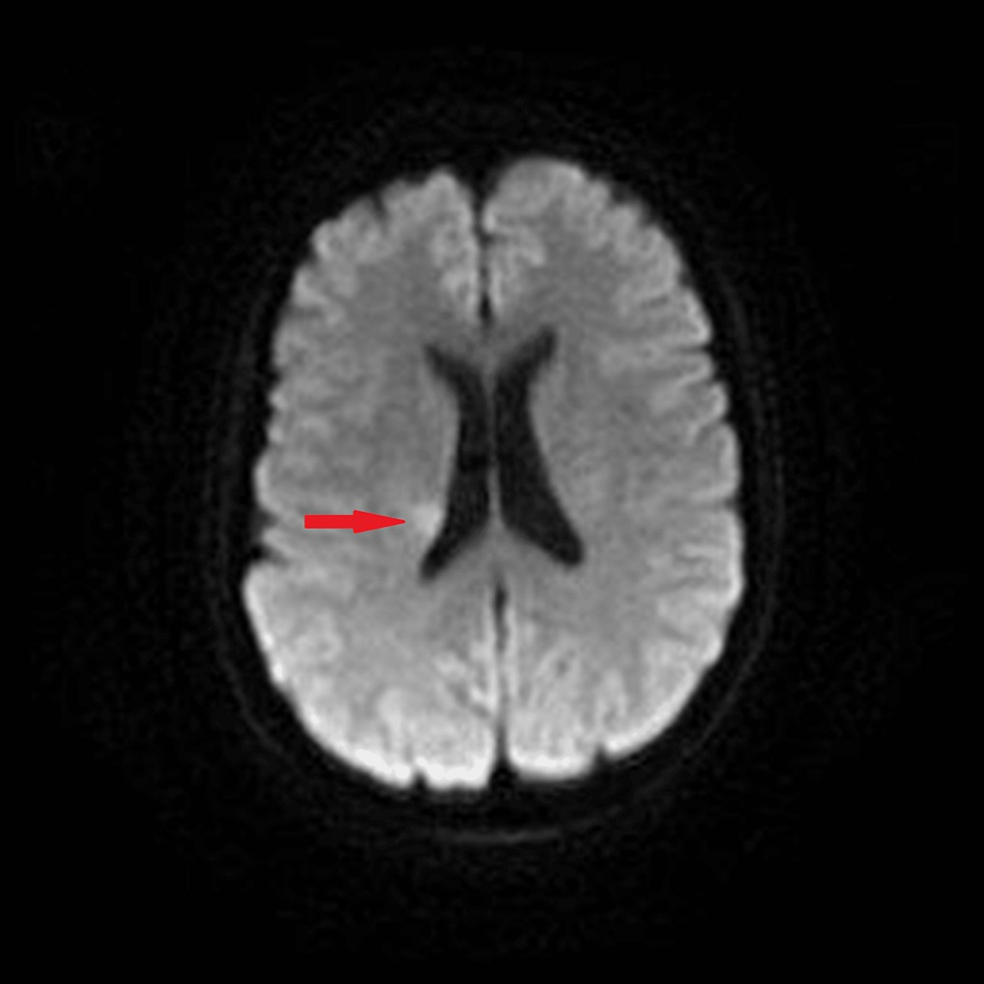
Diffusion-weighted magnetic resonance imaging scan of the brain Axial view demonstrating the subacute infarct in the right corona radiata (red arrow)
